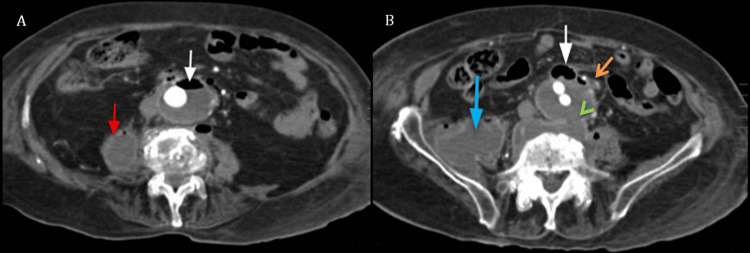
Axial slices of an abdominal CT angiography Image A. Demonstrates an aortic aneurysm with an air-fluid level on its anterior aspect (white arrow) and a collection within the right psoas muscle (red arrow). Image B. Highlights the same anterior air bubble (white arrow) in close proximity to a small intestinal loop at the duodenojejunal junction, adjacent to the aneurysm (orange arrow). The lateral collection extends along the trajectory of the psoas muscle (blue arrow). Additionally, an anterior paravertebral collection is noted, associated with a focal defect in the aortic wall directly in front of it (green arrowhead).
